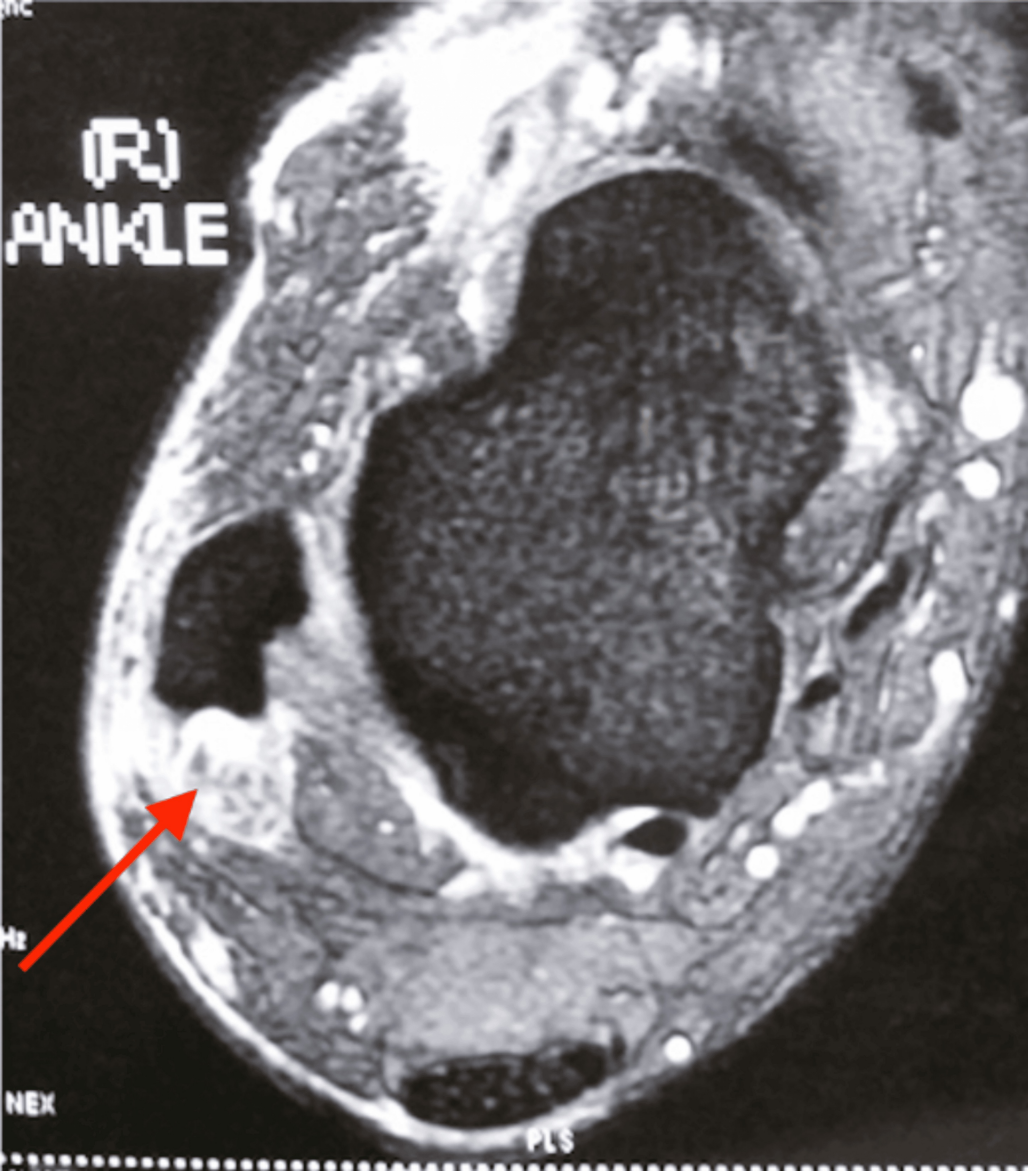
MRI axial cuts showing absent distal remnants of both peroneal tendons (red arrow).
